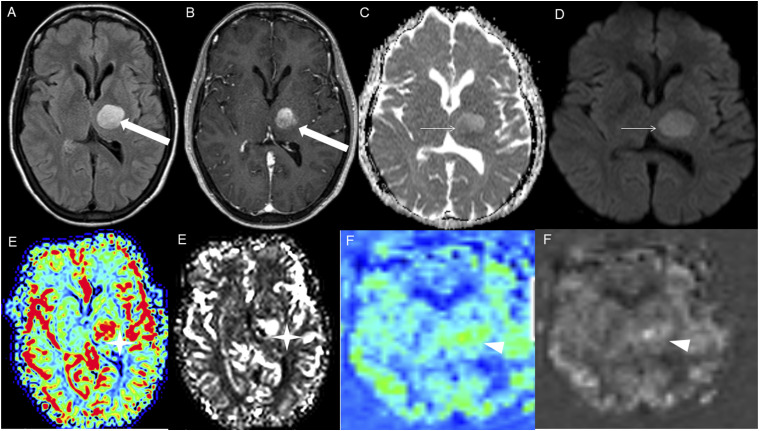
Axial FLAIR (A), post-contrast T1 weighted image (B), DWI (C), ADC map (D), dynamic susceptibility contrast DSC (E) and arterial spin labeling perfusion ASL (F), show a FLAIR hyperintense mass involving the left thalamus (A, thick white arrow) with heterogeneous post-contrast enhancement (B, thick white arrows). There is focal area of diffusion restriction medially on DWI/ADC map (C-D, thin arrows). There is elevated relative cerebral blood volume on DSC (E-star) and increased cerebral blood flow on ASL (F-arrowhead).
